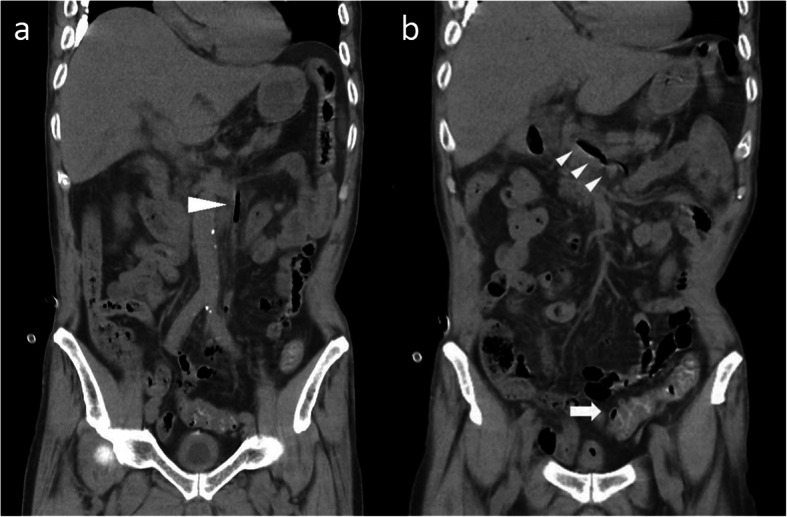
Nonenhanced CT at admission showed extensive thrombophlebitis of the portal venous system (a and b arrowhead) and sigmoid colon wall thickening with diverticulum (b arrow)
